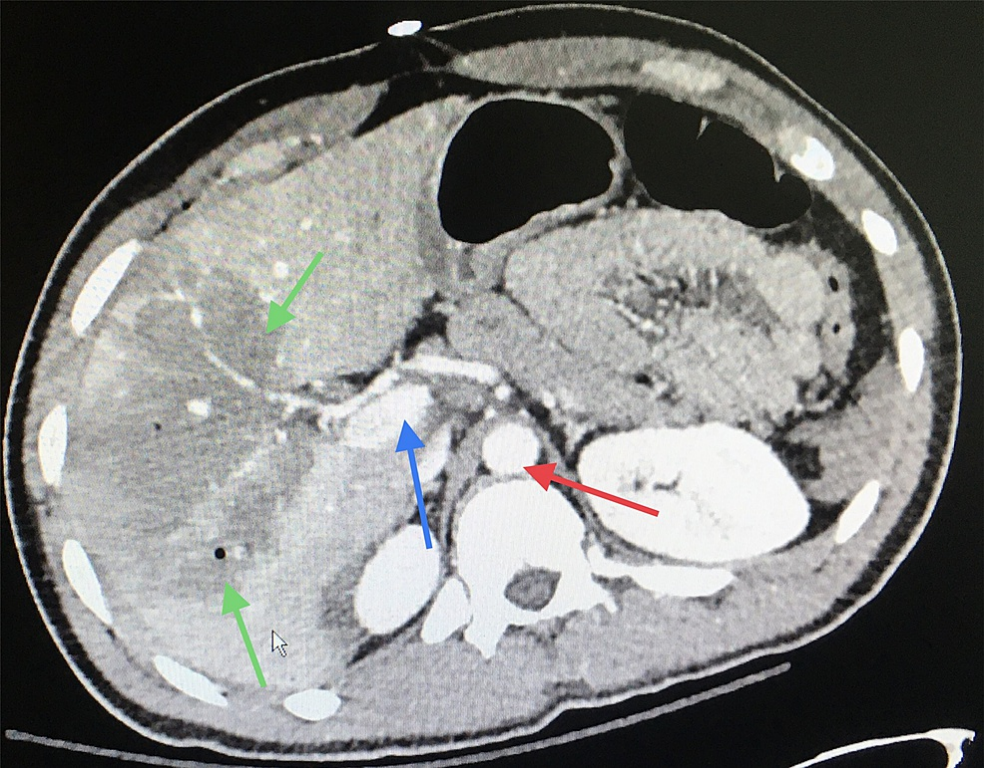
CT of the abdomen Green arrows show liver parenchymal disruption involving more than 25% of the right lobe with active bleeding (grade 4 liver injury), blue arrow shows extravasation of contrast consistent with active bleeding, and red arrow shows aorta color similar to the extravasation.
